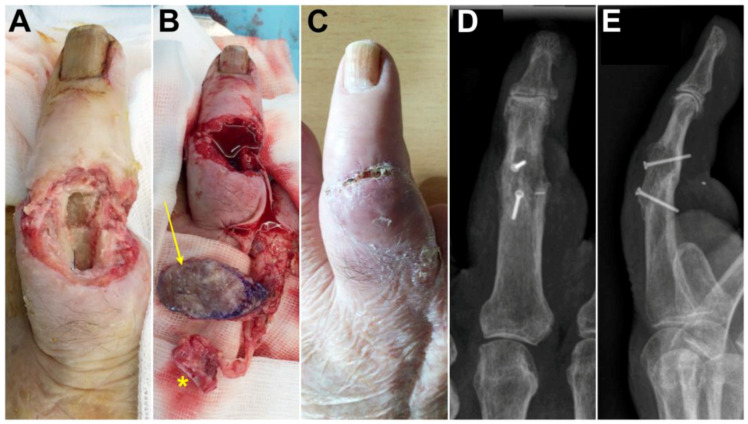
Case 5. (A) Photographs before surgery, dorsal aspect of the middle finger; (B) The elevated skin flap (arrow) and vascularized bone (asterisk); (C) postoperative photograph demonstrating a healed skin flap; (D,E) postoperative X-rays demonstrating fusion of the proximal interphalangeal joint.
